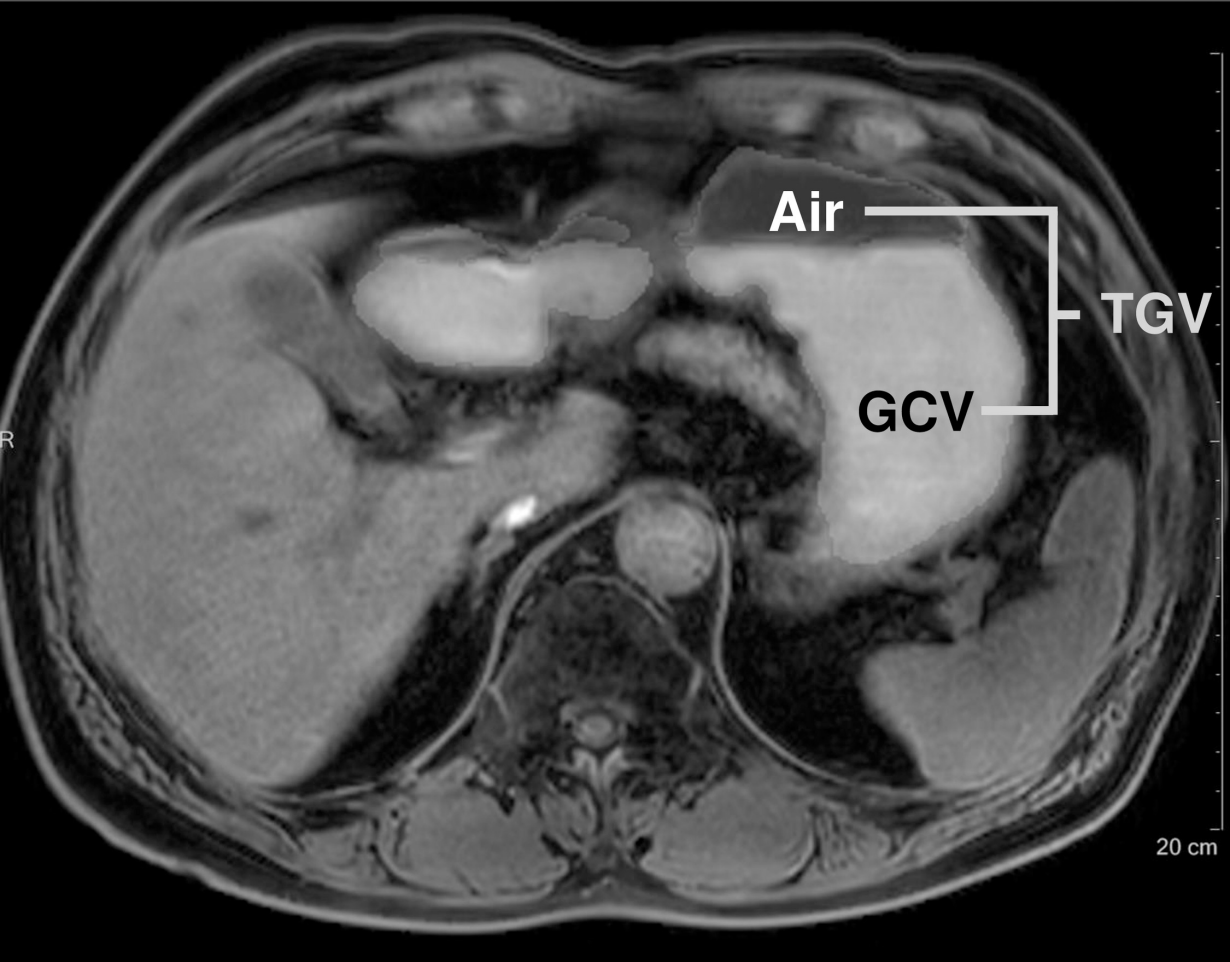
Gastric volume measurement of a 68-year-old man with Parkinson’s disease. In axial T1-weighted image, regions of interest (ROI) of gastric contents and air were drawn by a radiologist with volume analysis software. The volume of ROI was calculated automatically. TGV, total gastric volume, GCV, gastric content volume.
